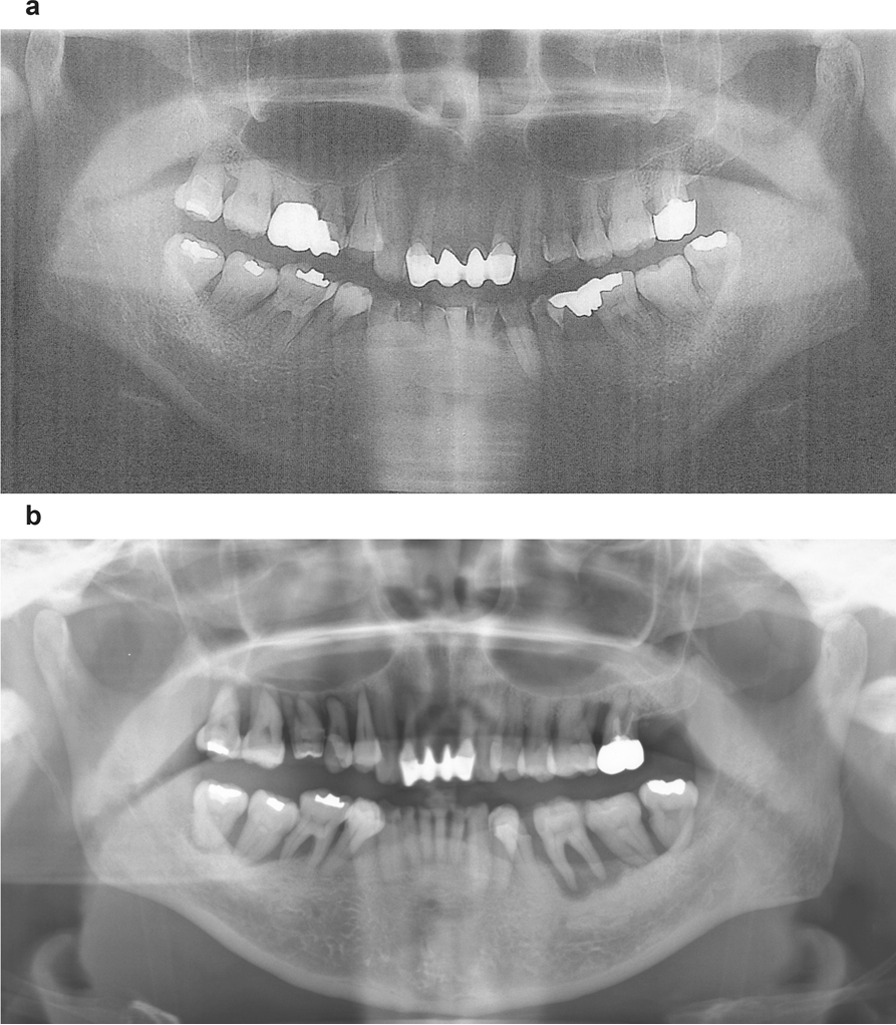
Panoramic oral radiographs reveal rapid alveolar bone loss after onset of Felty syndrome. a Panoramic radiograph taken 1 year and 5 months before the initial visit to our department. b Panoramic radiograph at the time of initial examination. Significant progression of alveolar bone resorption in a period of 1 year and 5 months is observed
